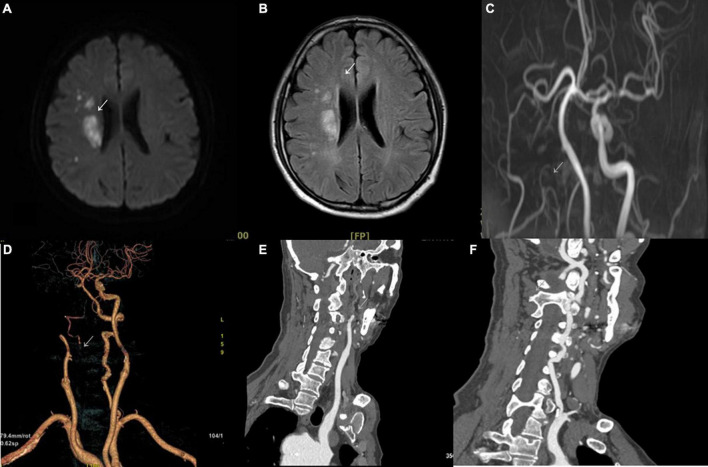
Brain MRI and CT angiography. (A) Diffusion-weighted imaging in the axial plane shows a high signal restriction in the right region beside the lateral ventricle (arrowhead) with a corresponding high signal in T2-weighted fluid-attenuated inversion recovery (B). (C) Multicategorization recommendation adjusting shows occlusion of the right internal carotid artery (arrowhead). The arterial phase of CT angiography also shows occlusion in volume rendering imaging (D) and in the sagittal plane (E) (arrowhead). (F) Stenosis of the initial segment of the left vertebral artery can be seen (arrowhead).
